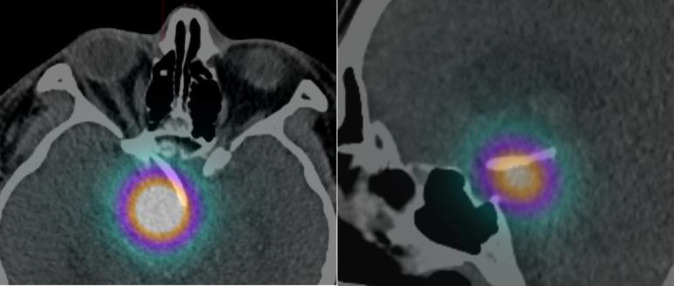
Post therapy Bremsstrahlung Yttrium-90 images using SPECT/CT. Tracer uptake was confined within the tumour with no evidence of leakage to the surrounding structures
